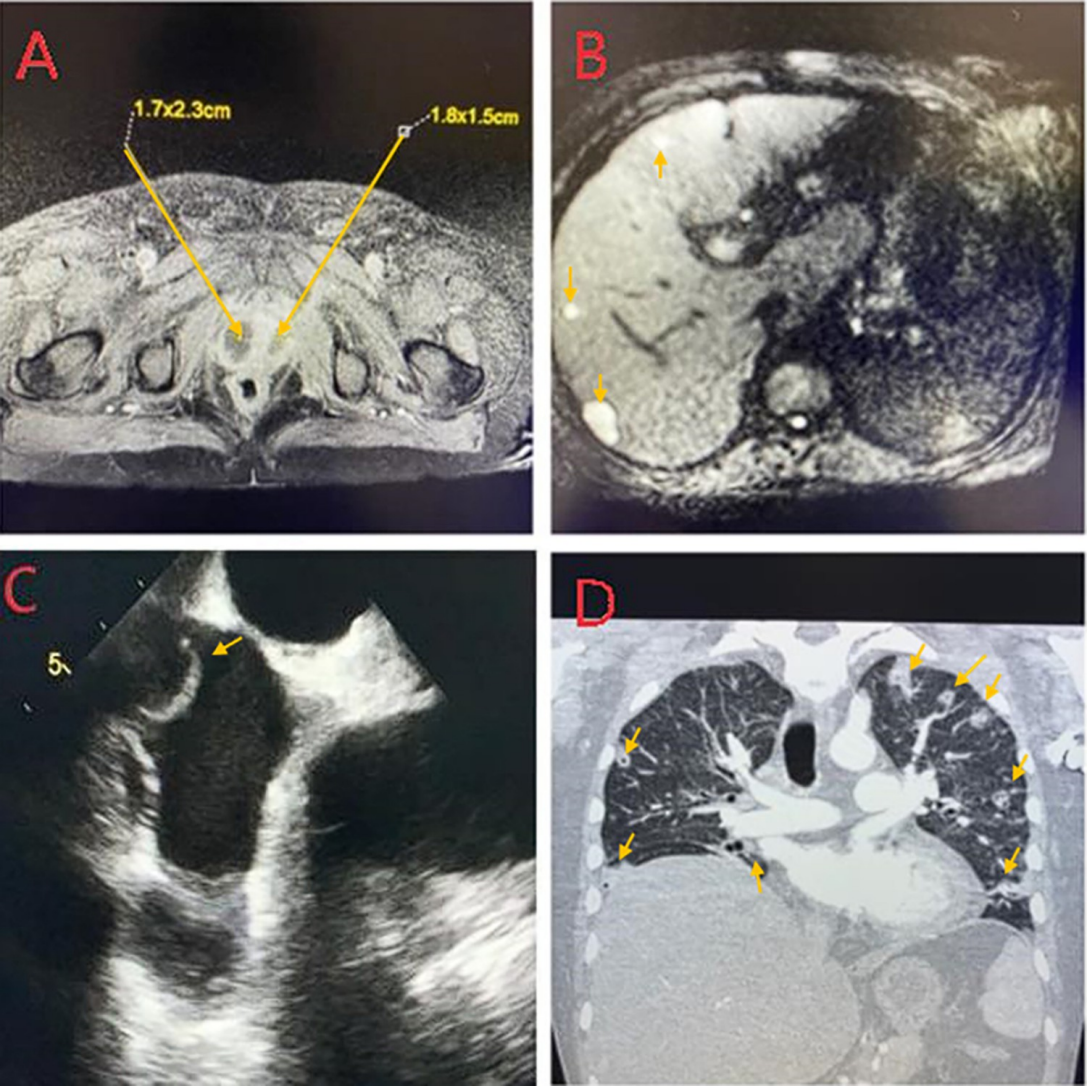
Metastatic septic infection by hypervirulent *Klebsiella pneumoniae* (2020CK-00441). Panel A: MRI Pelvis showing bilateral prostatic abscesses measuring 1.7x2.3cm on right and 1.8x1.5cm on left. Panel B: MRI Abdomen series showing multiple small hepatic abscesses throughout the liver. Panel C: Trans-esophageal echocardiogram showing a clot traversing the right atrium from the inferior vena cava. Panel D: CT imaging of chest showing multiple septic emboli throughout bilateral lung fields. Pathology identified by yellow arrow in each panel.
